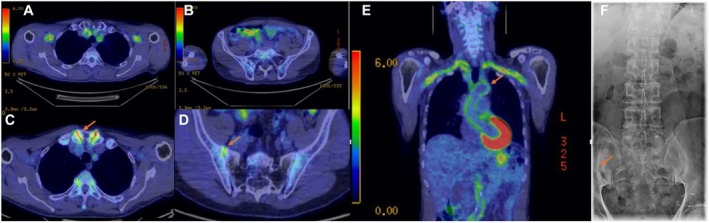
Imagesof a patient with polymyalgia rheumatica, giant cell arteritis, and spondyloarthritis. A 61‐year‐old male patient presented with polymyalgia rheumatica symptoms, chest pain, and constitutional symptoms. PET/CT revealed extensive involvement of the aorta, subclavian, and carotid arteries. High‐dose steroid therapy was initiated and subsequently tapered, and the patient was observed under methotrexate treatment. The patient reported low back pain since the initial presentation. A follow‐up PET/CT scan performed after four years revealed sacroiliitis. (A and B) Initial PET/CT showed no significant sacroiliac or costovertebral joint involvement. (C and D) PET/CT at year four demonstrated sacroiliac, sternoclavicular, and costovertebral joint involvement. (E) Initial PET/CT revealed extensive aortic and subclavian involvement. (F) Sacroiliac radiograph showed bilateral sclerosis (more extensive on the right side) and bilateral stage 2 sacroiliitis based on the modified New York criteria. PET/CT, positron emission tomography/computed tomography. Color figure can be viewed in the online issue, which is available at http://onlinelibrary.wiley.com/doi/10.1002/art.43320/abstract.
